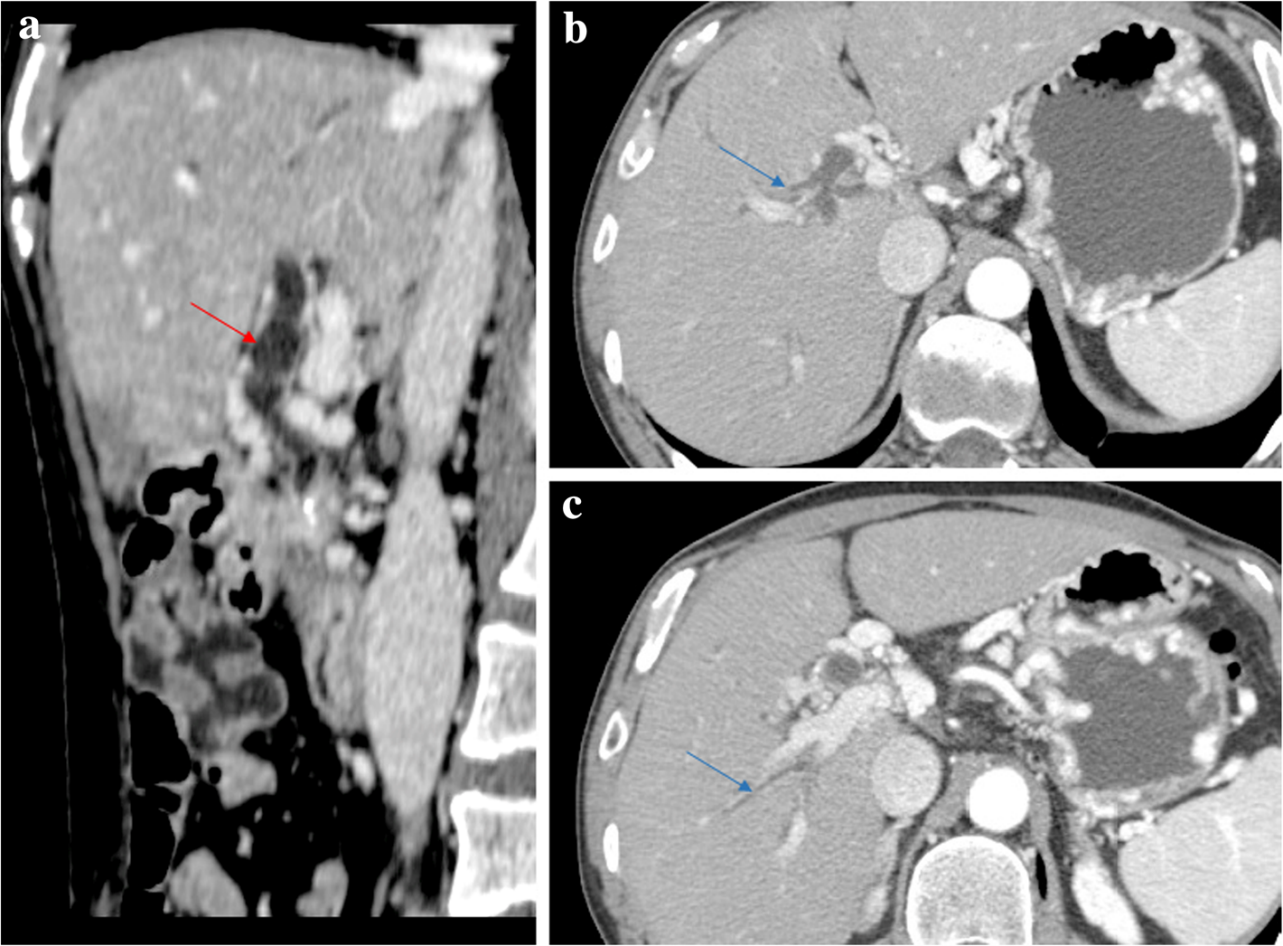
Portal biliopathy. Sagittal portal venous phase CT images shows tortuous common bile duct (red arrow) encircled by portal cavernoma, leading to dilation of peripheral bile ducts (blue arrows). Note also gastric varices due to PH
